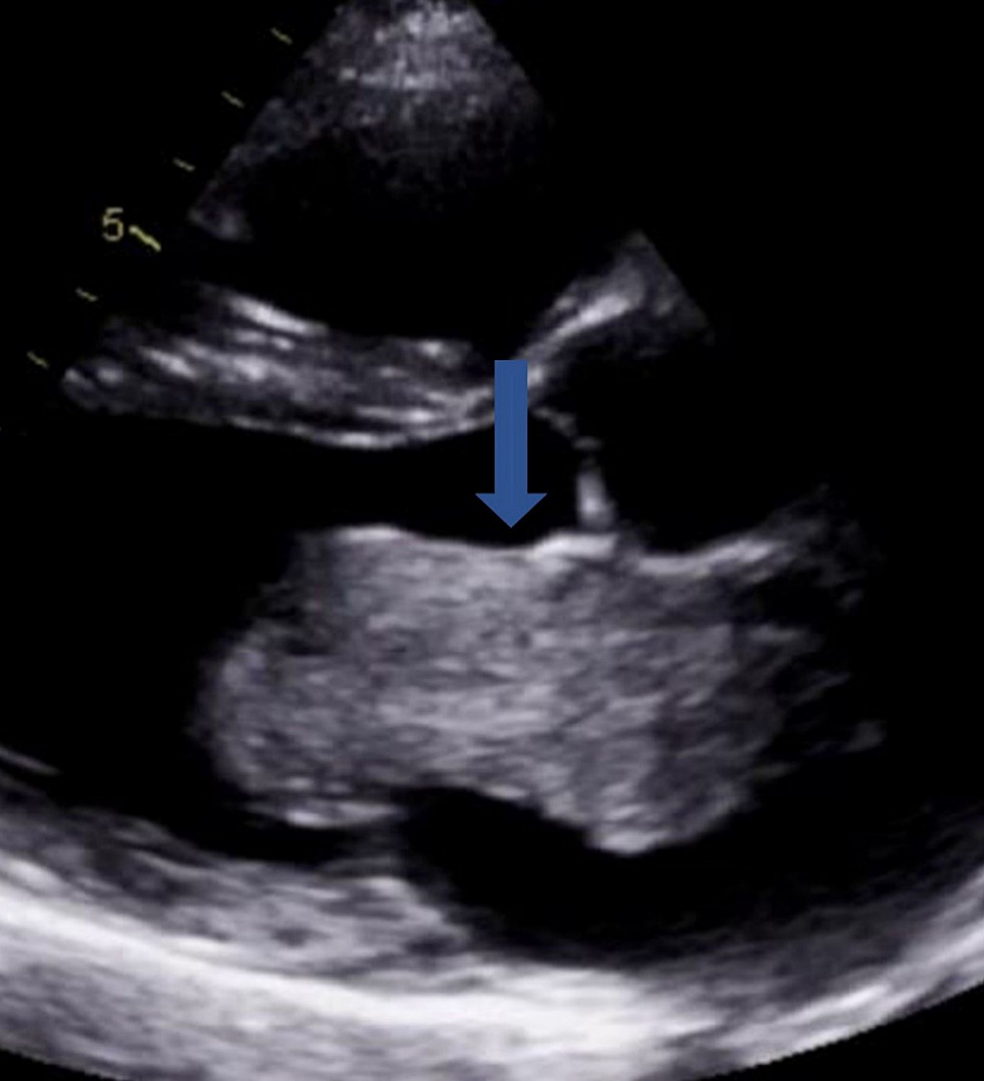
Echocardiography parasternal long-axis view showing left atrial myxoma
